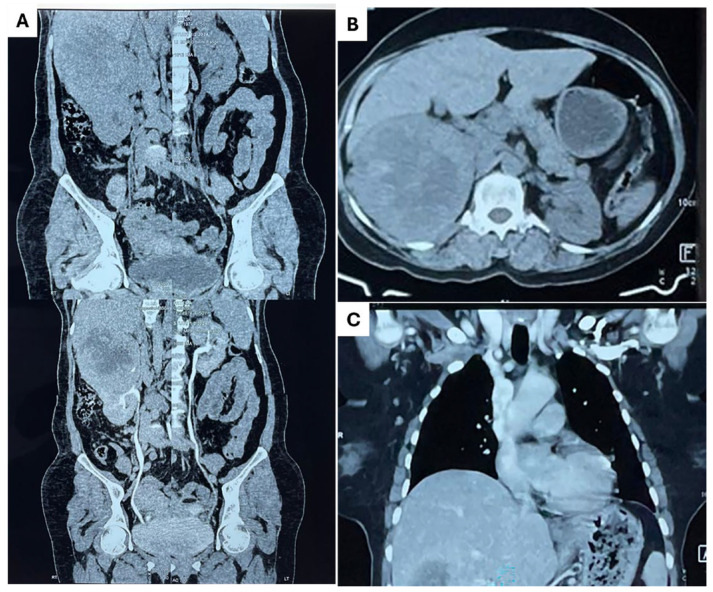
CT urogram and CT scan of the lung. (A,B) The CT urogram of the kidney showed a large, heterogeneous, calcified mass with mixed density and enhancement in the upper pole of the right kidney, along with an additional mass in the left adrenal area. (C) The chest CT scan revealed metastatic lesions in the lungs.
